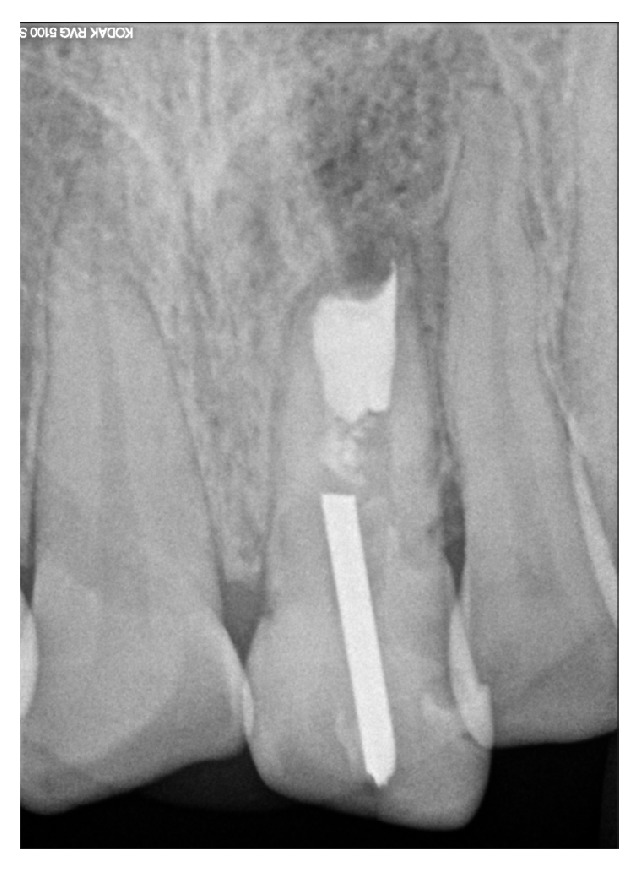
A follow-up radiograph at 52 months (4 years and 4 months) reveals complete healing of the initial periapical bone defect. At this time, the case was considered to be healed.
